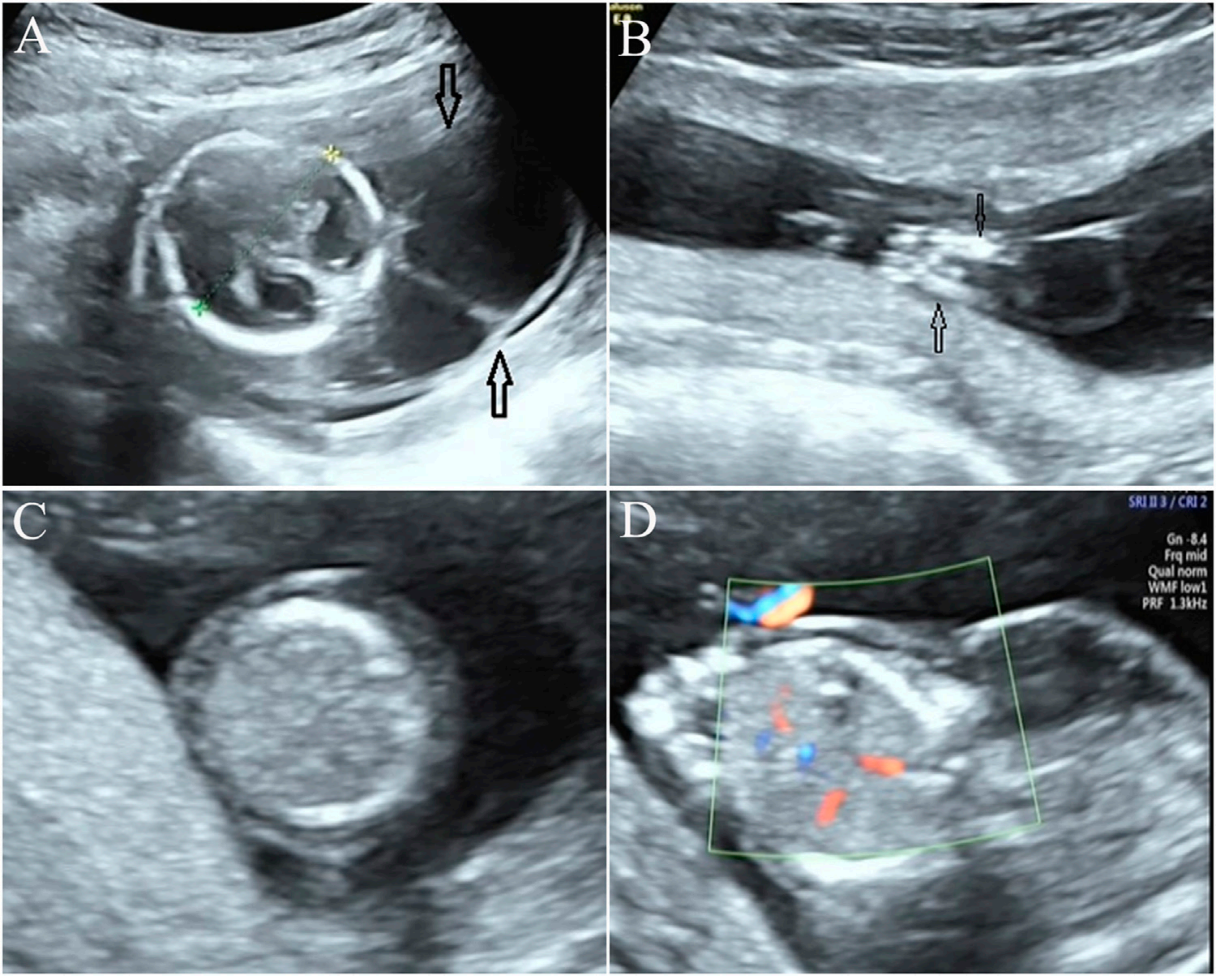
Prenatal ultrasonic examination results of the fetus. (A) Ultrasound examination elicited neck water sac tumor in the fetus. (B) Approach, flexion, and fixation of both lower limbs were observed in the fetus, indicating joint contractures. (C,D): the trunk skin layer is obviously thickened, and the echo is reduced, and diagnosed as fetal edema.
